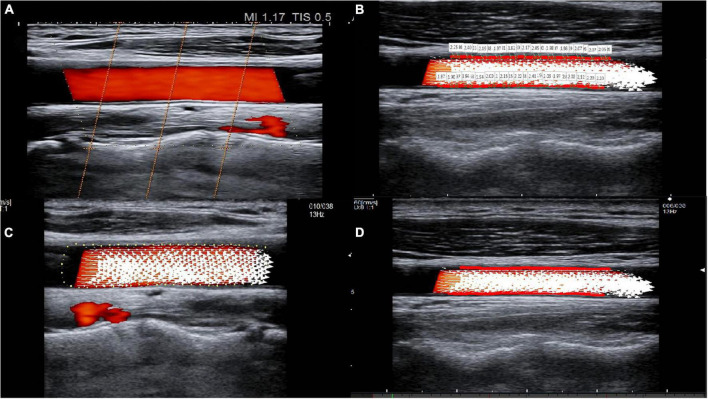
The visualization of common carotid artery blood flow and the quantitative calculation of WSS. (A) Three sound beams intersecting with the color Doppler appear when the VFM button is activated. Cross-beam vascular VFM creates a starting point to replace the vessel wall, and it obtains blood flow velocity information perpendicular to the direction of the color Doppler. (B) Tracing of the intima of the anterior and posterior walls of the common carotid artery and visualization of the WSS at each point of the anterior and posterior walls of the carotid artery. (C) The white arrow represents the blood flow velocity vector of the carotid artery. (D) The red line(s) on the vessel wall indicates that the WSS is high.
